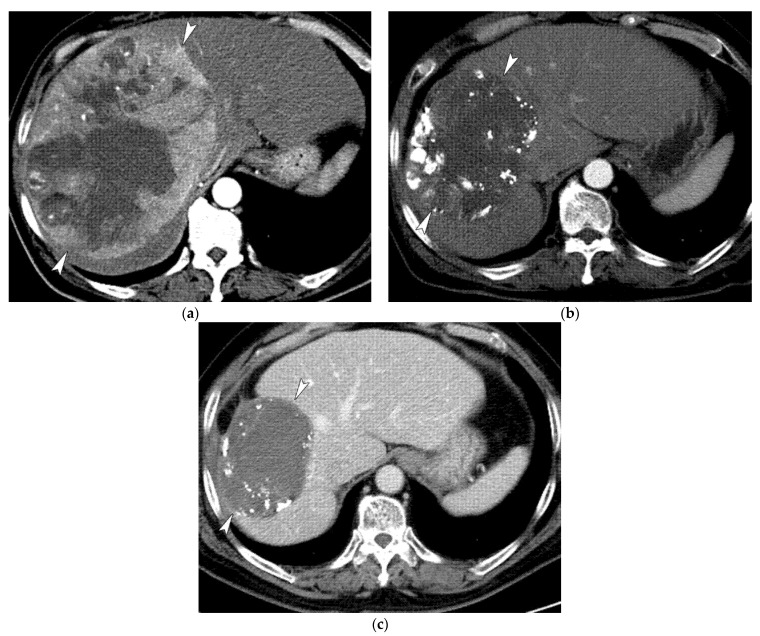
Images of a 64-year-old male patient with HCC. (a) Contrast-enhanced axial CT image in the arterial phase shows a huge enhancing mass (19 cm in maximal diameter; arrowheads). (b) CT at 1 year after five sessions of TACE shows necrotic change with lipiodol uptake in the tumor and a decrease in tumor size (13 cm; arrowheads). (c) CT at 5 years after eight sessions of TACE shows a further decrease in tumor size without recurrence (10 cm; arrowheads).
